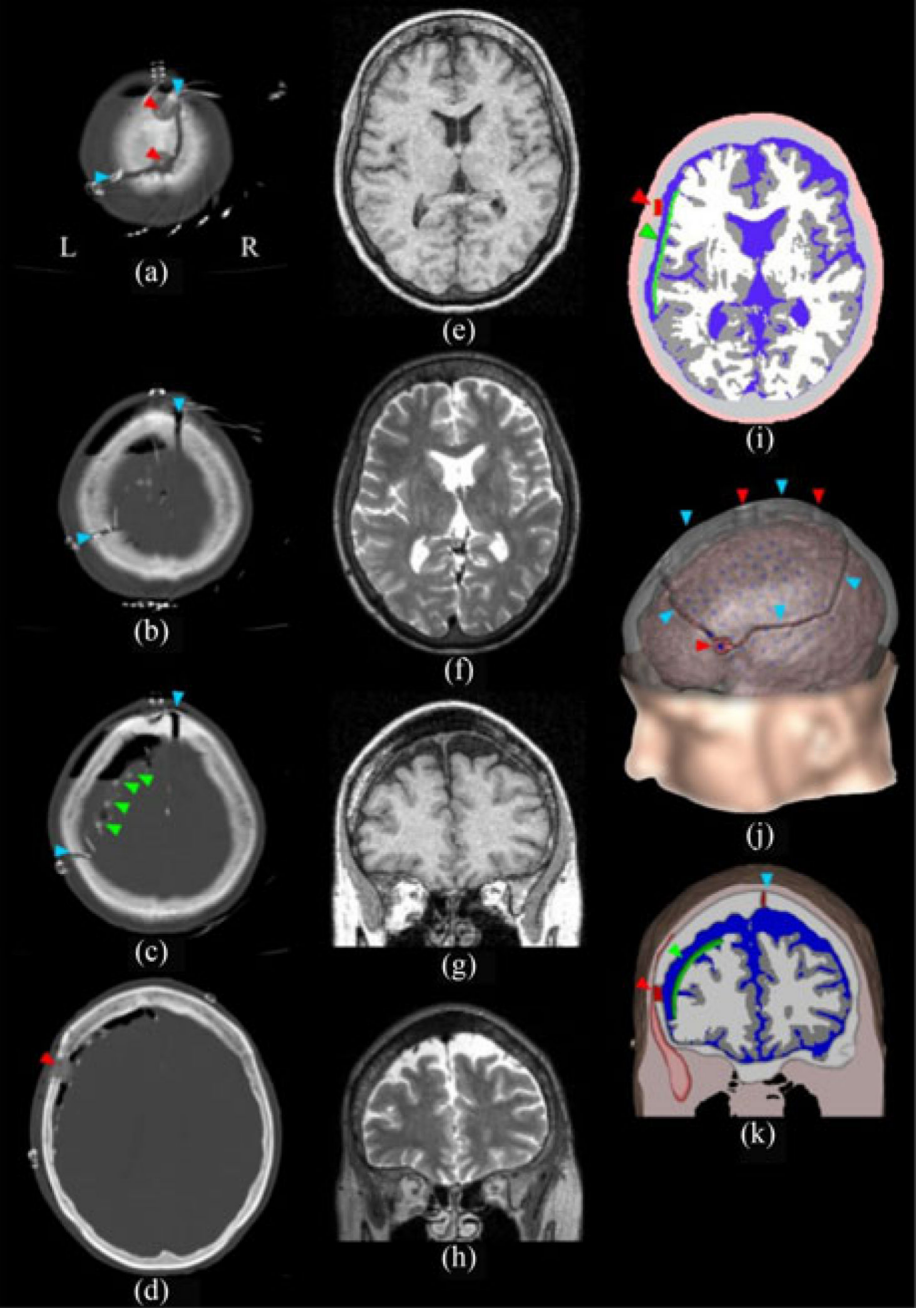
CT and MRI imaging data and volume conductor head model. (a)–(d) Axial CT images taken after subdural electrode implantation. (e) and (f) Axial slices through preoperative T1 and T2 weighted MRI data, respectively. (g) and (h) Coronal slices through preoperative T1 and T2 weighted MRI data, respectively. (i) Axial slice through segmented data of Head Model 1 (HM 1, with craniotomy defects and with grid, see Section II). For comparison with the MRI data the slice was taken at the same position as in (e) and (f). Soft tissue: light pink; air: black; temporalis muscle: dark pink; skull: light gray; craniotomy defects: red; ECoG grid: green; CSF: blue; gray matter: dark gray; and white matter: light gray. (j) 3-D visualization of HM 1. Gray matter surface: pink; electrodes: blue; skull: transparent gray. (k) 3-D coronal slice through volume conductor model (HM1). For comparison with the MRI data, the slice was taken at the same position as in (g) and (h). Conventions as in (i). The red, turquoise, and green arrowheads indicate the burr holes, saw lines, and the electrode grid, respectively.
